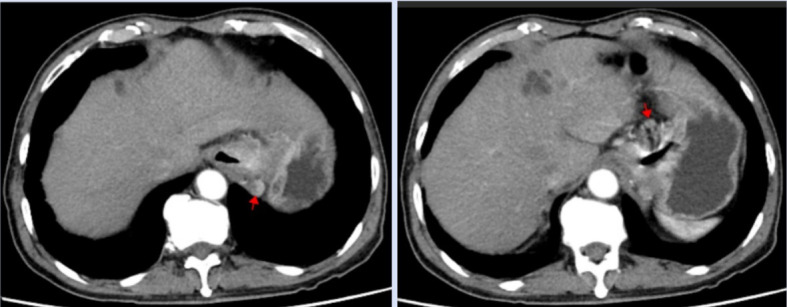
CT scan revealed multiple enlarged lymph nodes around the stomach.
